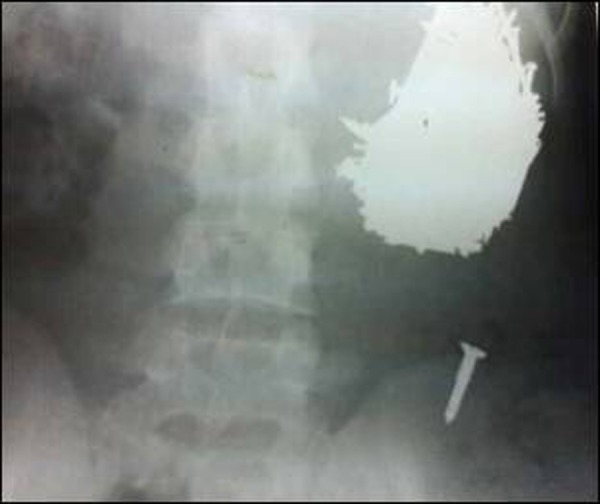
Figure 1: X-ray showing metal objects in the hugely distended stomach.
